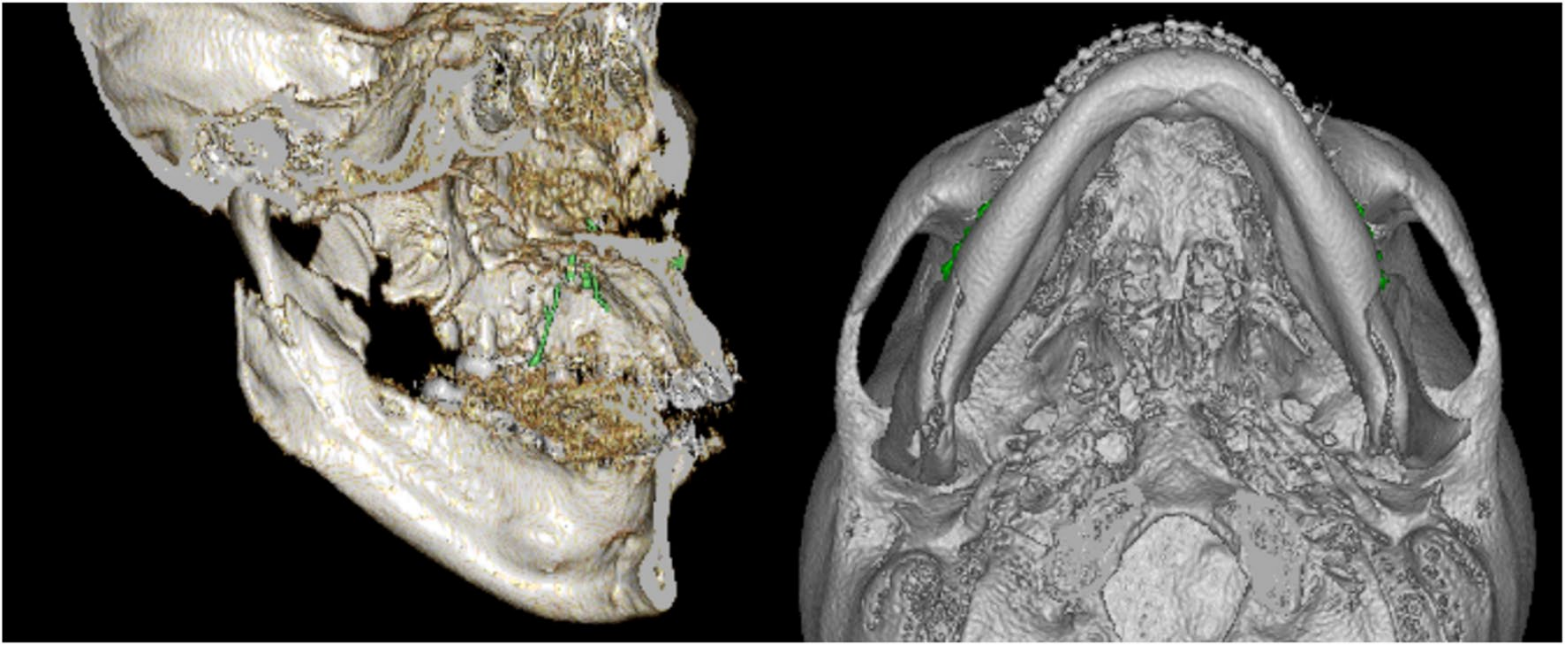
CT images showing LS. A horizontal fracture line extending to the posterior border of the ramus can be seen
